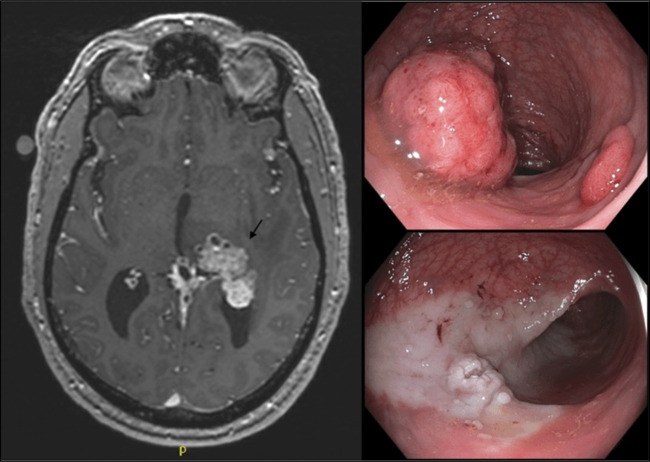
Brain MRI demonstrating metastasis to the left thalamus (black arrow). On the right, colonoscopy images showing a fungating mass in the rectum and postsnare colon. MRI, magnetic resonance imaging.
